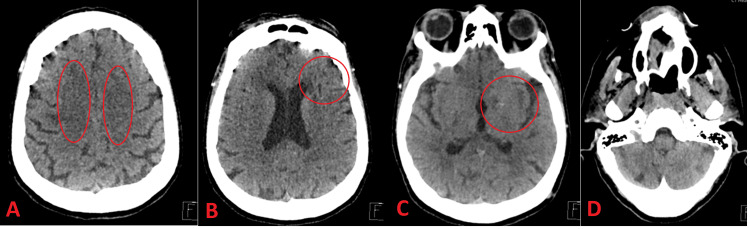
Noncontrast CT head demonstrating extensive chronic microvascular changes and an old infarction in the right cerebellum The CT scan shows bilateral periventricular white matter ischemic changes consistent with small vessel disease. A: Bilateral periventricular low‐attenuation areas consistent with chronic small‐vessel ischemic changes. B: Ongoing white matter changes, again suggestive of microvascular ischemia and more evident in the right frontal region. C: Extension of ischemic changes in the deep white matter; could reflect chronic lacunar infarcts or gliosis. D: Right cerebellar volume loss and encephalomalacia consistent with an old infarction.
